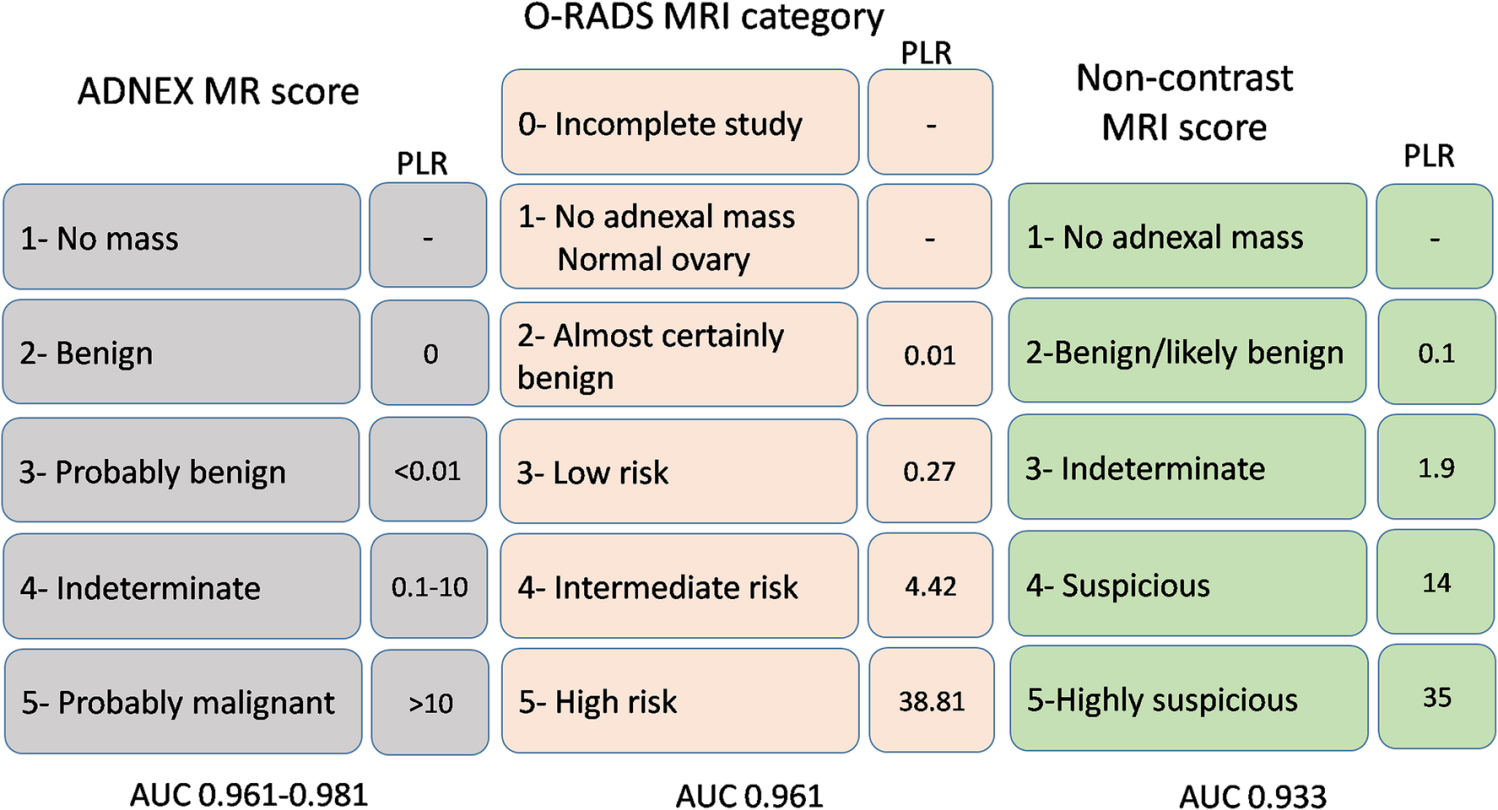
Comparison of ADNEX MR score, O–RADS MRI category and proposed *non-contrast MRI score*. The area under the curve for ADNEX MR score refers to AUCs according to different readers in the training set. *AUC* area under the curve, *MR(I)* magnetic resonance (imaging), *O–RADS* Ovarian–Adnexal Imaging–Reporting and Data System, *PLR* positive likelihood ratio
